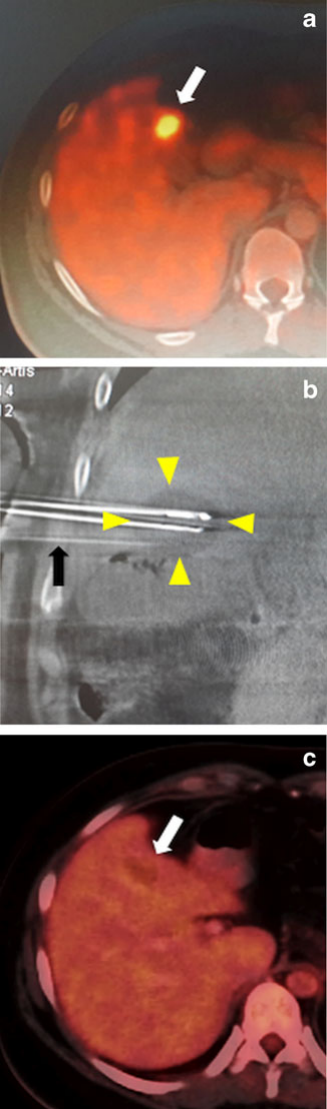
Cryoablation in the liver. a The axial PET-CT image shows a subcapsular FDG-avid lesion (*white arrow*). The patient had previously undergone surgery for cholangiocarcinoma, and the biopsy showed the lesion to be metastatic cholangiocarcinoma. b Cryoablation was performed under US and cone-beam CT guidance, which shows the iceball (*arrowheads*) produced by two cryoneedles covering the lesion. Another needle (*black arrow*) was also inserted for fluid injection to separate the adjacent transverse colon from the tumour. c One year later, the control PET-CT image shows complete metabolic response in place of the lesion
